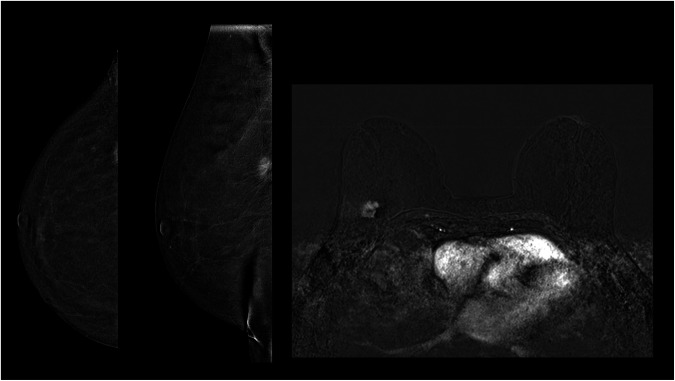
Posteriorly located breast cancer with limited assessment at CEM. CEM shows an enhancing lesion in the posterior right breast, with incomplete evaluation of the posterior extent and chest wall relationship. Subsequent breast MRI allowed more accurate assessment of tumour extent and exclusion of chest wall involvement, guiding surgical planning
